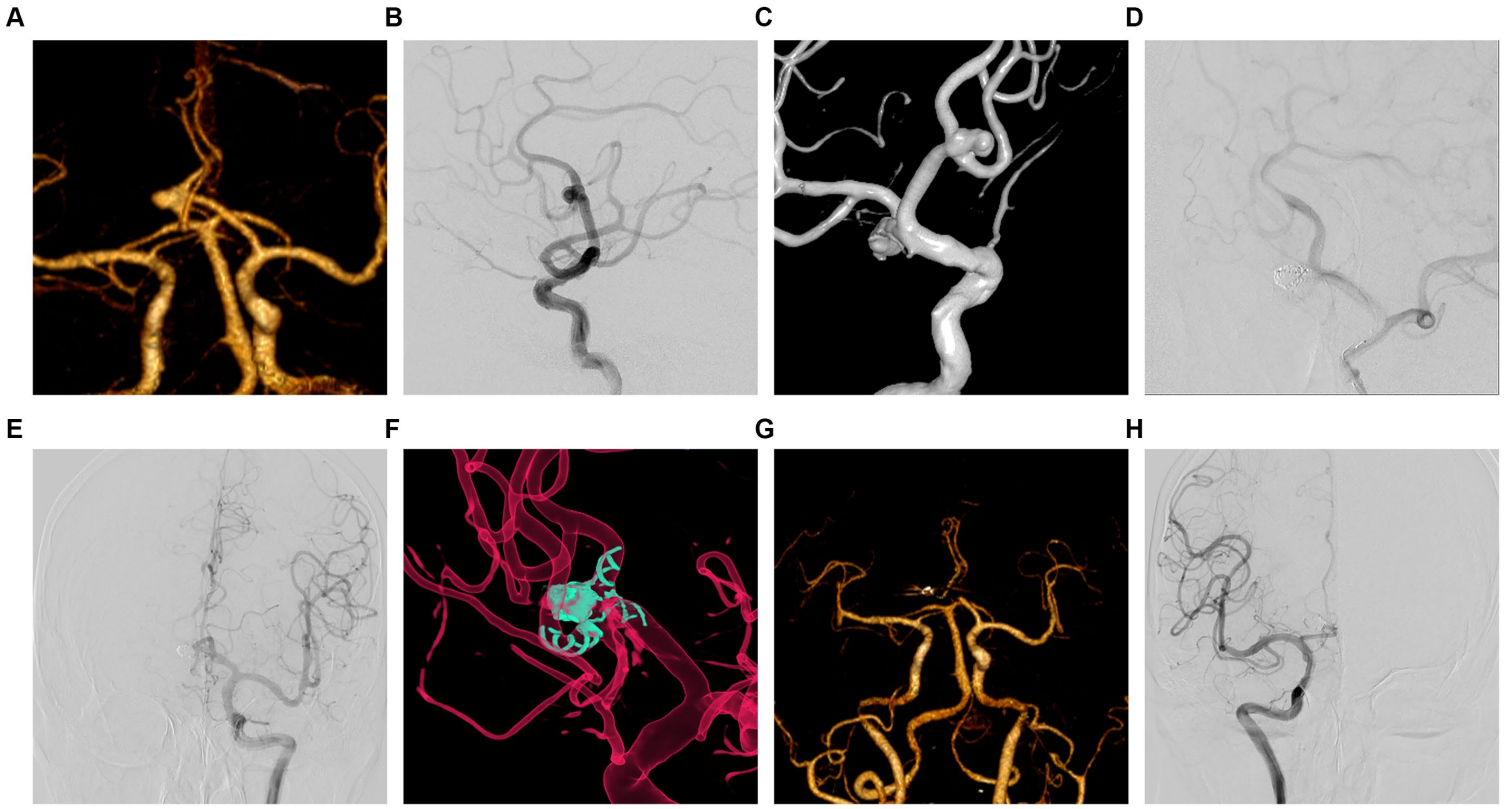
Intraoperative complications and postoperative follow-up period for embolization of unruptured AcomA patient. (A) A 67-year-old male, diagnosed with an AcomA aneurysm by CTA. (B) Cerebral angiography in the lateral position clearly showed an aneurysm in the AcomA. (C) 3D reconstruction clearly showed that the aneurysm in the AcomA involved both anterior cerebral arteries, and Y-configuration stent-assisted embolization was proposed for the treatment. (D) The right ACA was not visualized by anterior and lateral position angiography during the procedure. (E) Immediate administration of tirofiban was conducted through a guiding catheter and microcatheter, with a gradual sequential injection totaling 23 mL over 3 min. Simultaneously, a peripheral intravenous infusion was maintained at a dosage of 0.1 μg/kg/min. Following the completion of the injection, a subsequent lateral angiography demonstrated successful reperfusion of the anterior cerebral artery. (F) Bilateral ACA was well illuminated by 3D reconstruction in immediate postoperative angiography. (G) The bilateral ACA was well-luminated by CTA one week after surgery. (H) The right ACA was well-illuminated by cerebral angiography at 10 months after surgery.
